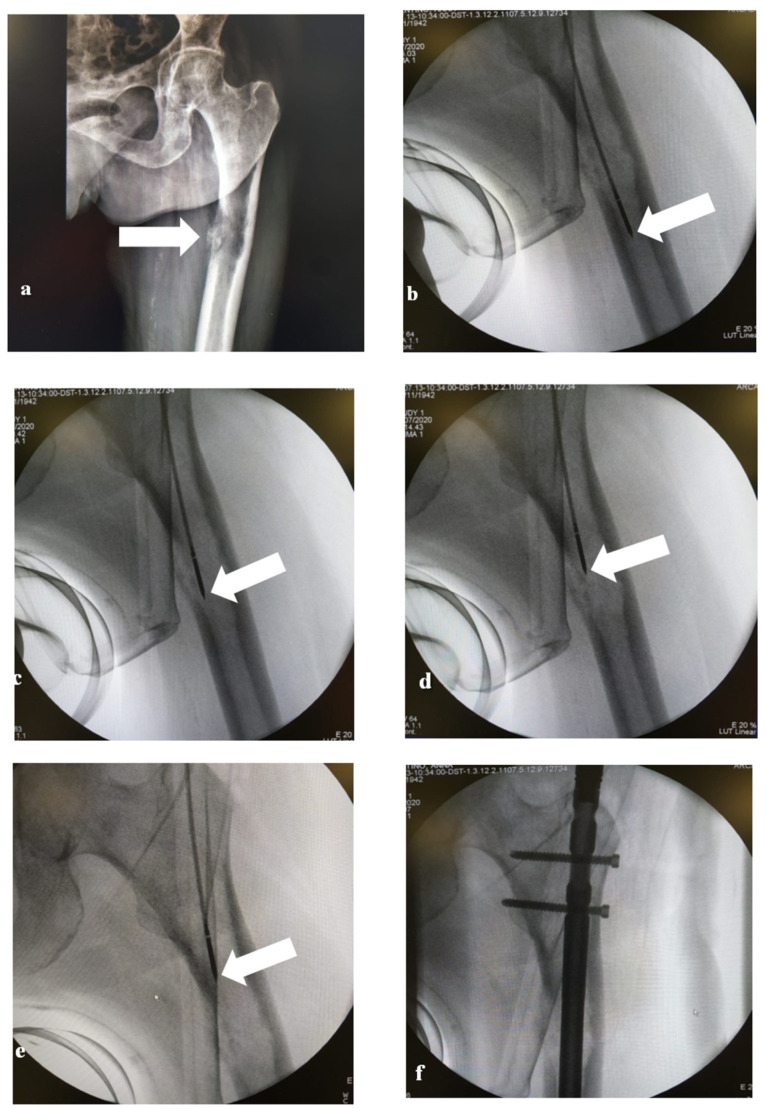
A 54-year-old female patient with a left femur metastasis from breast cancer. The X-ray (a) shows a lytic lesion of the left femur with erosion of the medial cortical profile. The C-Arm images (b–e) show 4 different positions of the MW antenna from distal to proximal edge of the lytic lesion. The C-Arm image (f) shows the presence of the endoprosthetic replacement.
